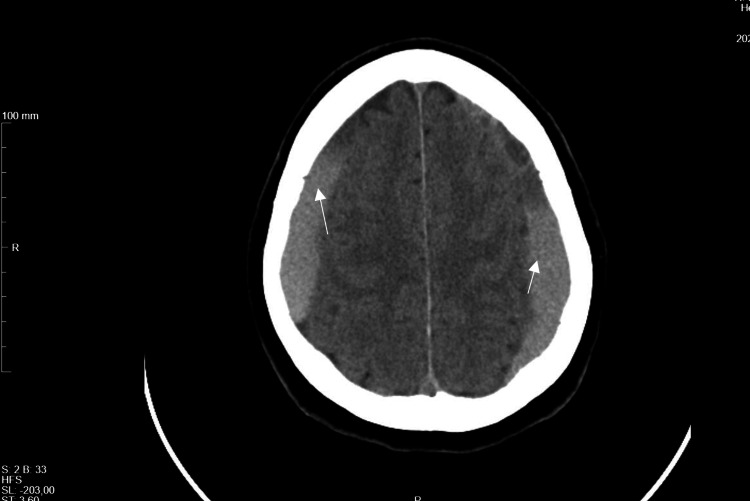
CT-scan showing bilateral frontoparietal hypodense subdural collections Arrows: subdural collections
